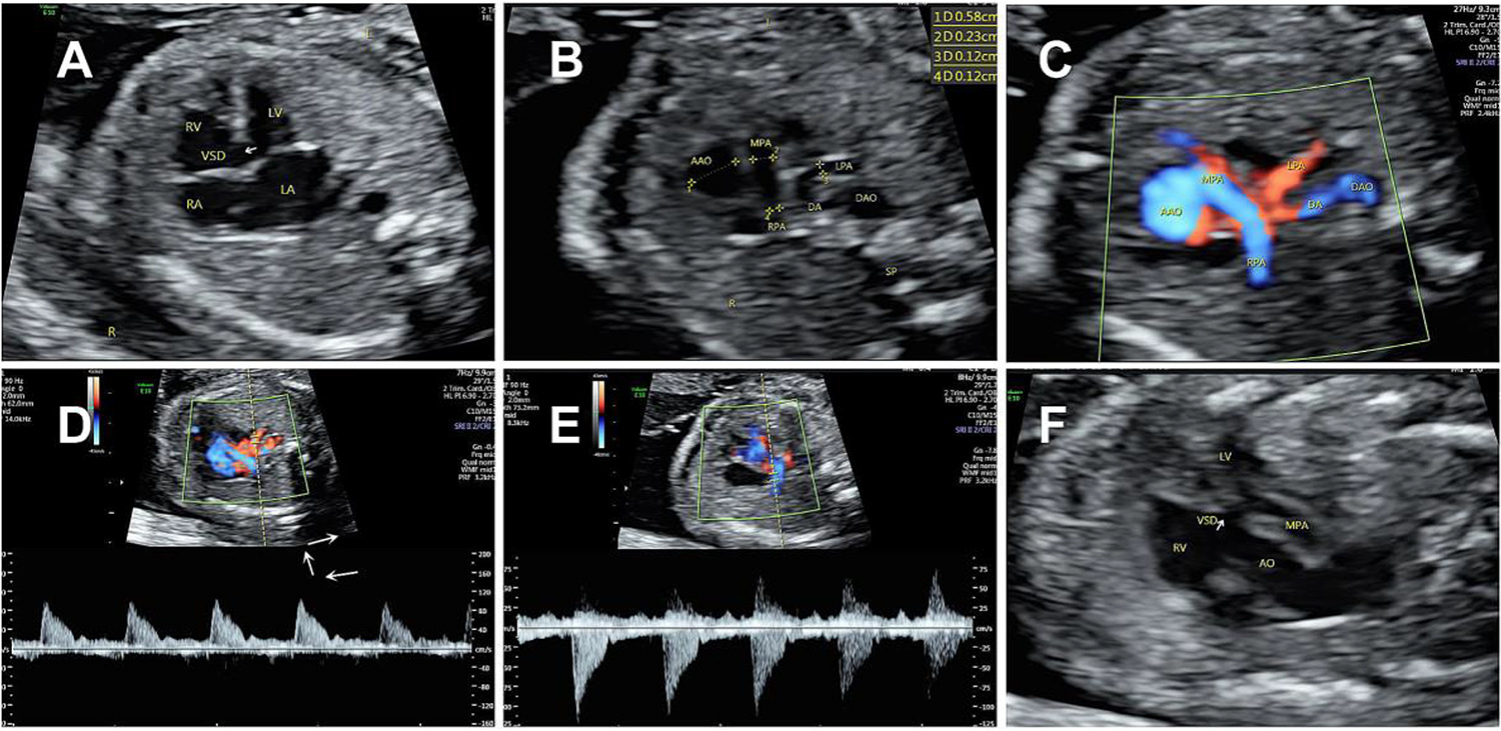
Prenatal echocardiogram showing (A) a large ventricular septal defect. (B,C) Main pulmonary artery (MPA-2.3 mm) only gave rise to the right pulmonary artery (RPA-1.2 mm). CDFI showed a blood flow (LPA-1.2 mm) originated from the descending aorta through the ductus arteriosus to supply the left pulmonary artery. (D,E) Spectral Doppler ultrasound showed similar high resistance waveforms of the pulmonary arteries on both sides. (F) A complete transposition of the aorta originating solely from the right ventricle.
